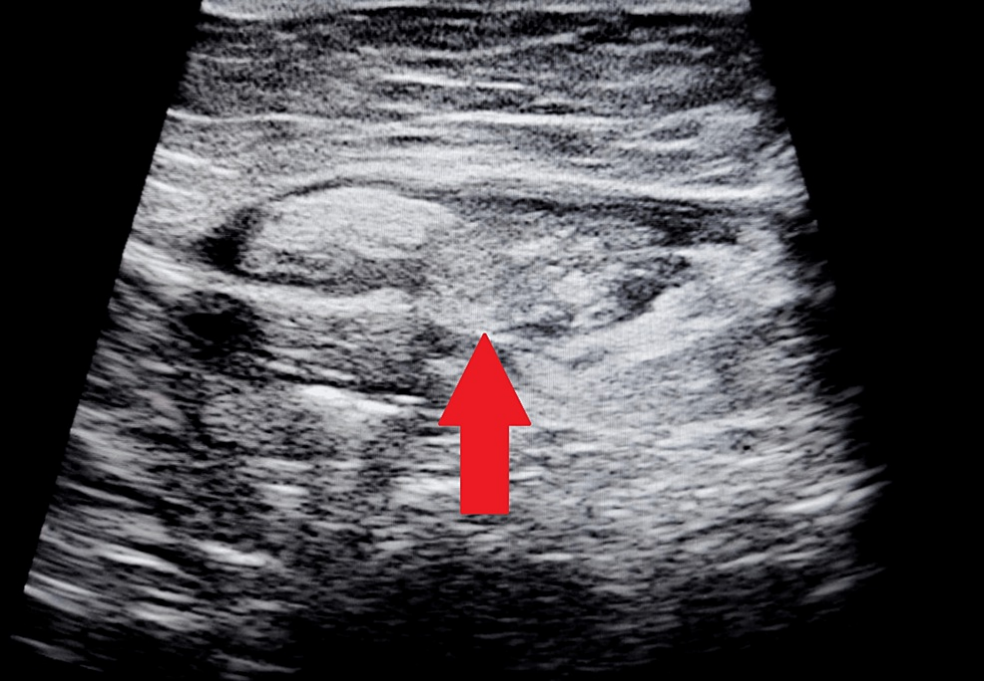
Ultrasound image showing hemoperitoneum.
